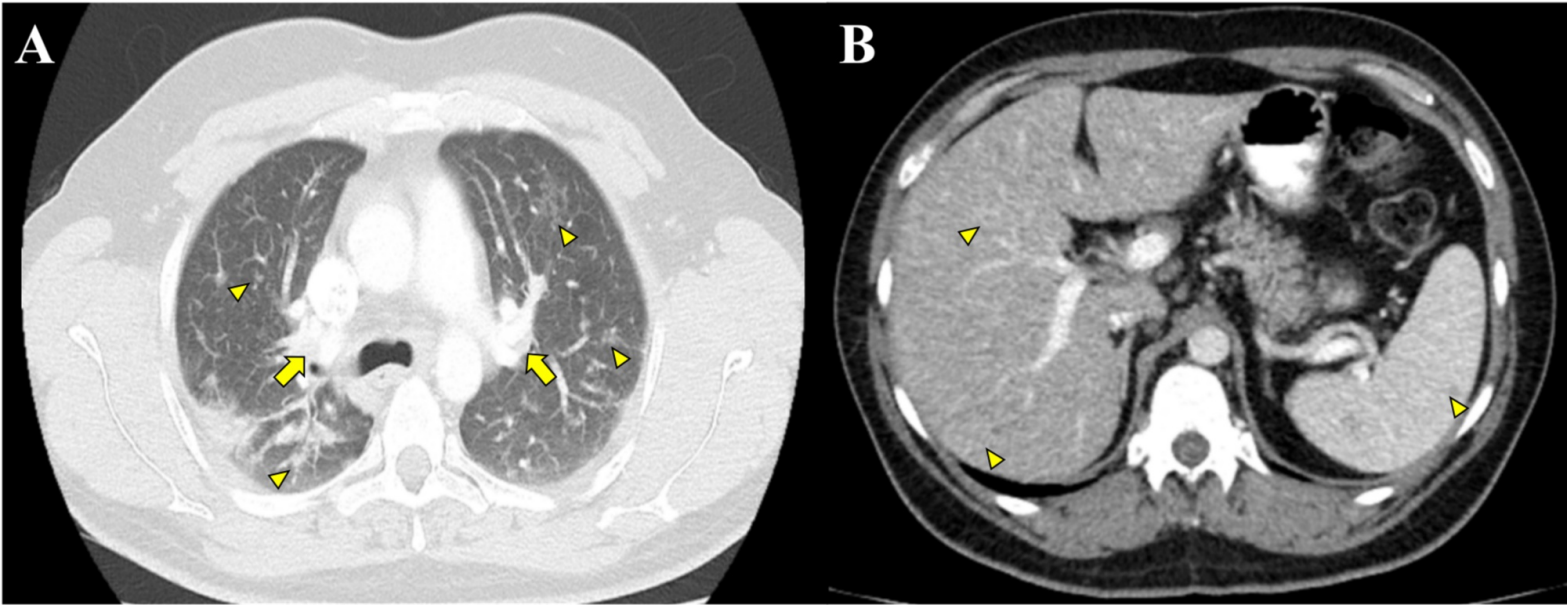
Whole-body computed tomography. (A) Enlargement of hilar lymph nodes (arrows) and micronodular pattern (arrowheads). (B) Diffuse hepatic and splenic involvement (arrowheads).
